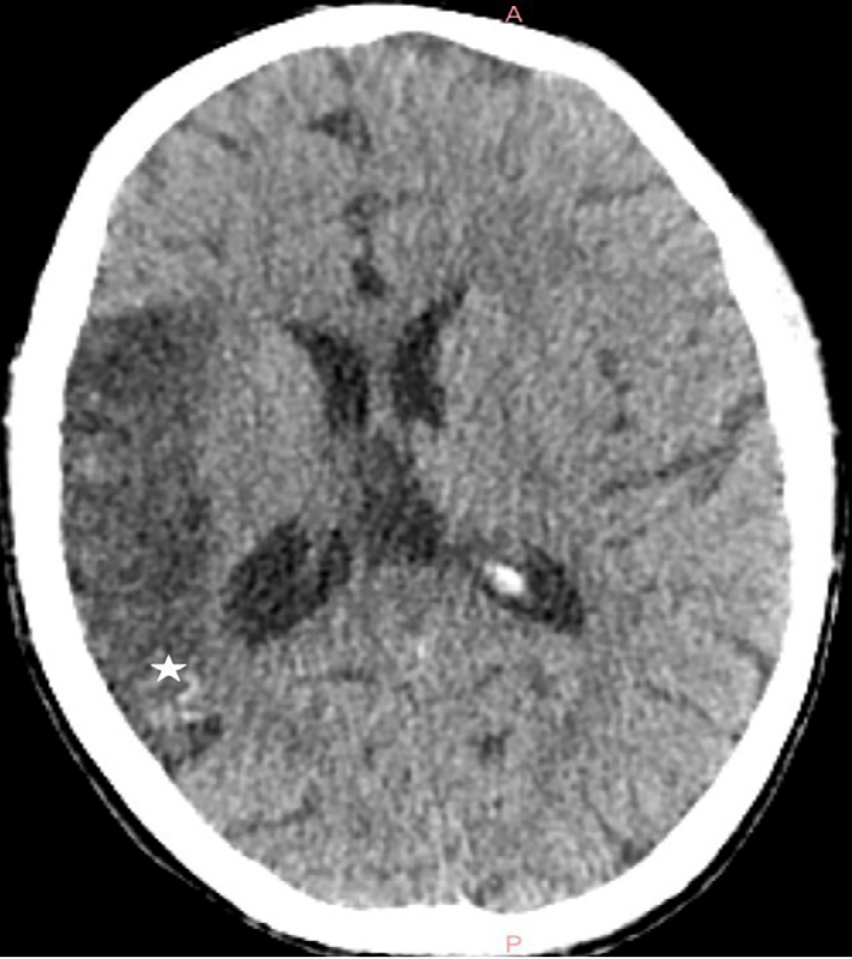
Axial noncontrast brain CT in Sturge-Weber syndrome type III The image shows right cerebral hemihypotrophy with spontaneous cortical hyperdensities corresponding to fine calcifications (*). These gyriform calcifications are characteristic of SWS and represent chronic hypoxic injury to the cortex.
